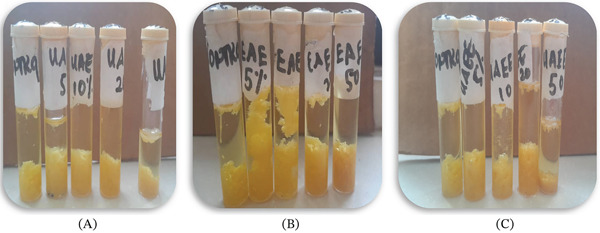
Images of mayonnaise after freezing at −20°C and thawing at 25°C for 30 min. (A) Mayonnaise made with proteins from ultrasound‐assisted extraction (UAE), (B) mayonnaise made with proteins from enzyme‐assisted extraction (EAE), and (C) mayonnaise made with proteins from ultrasound‐assisted enzymatic extraction (UAEE). Starting from the left, the control is followed by 5%, 10%, 20%, and 50% substitution.
